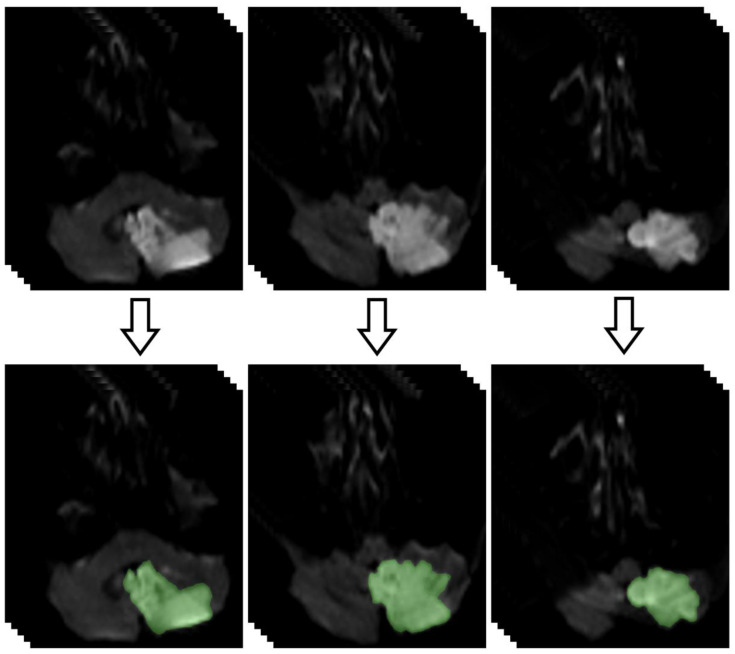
DWI images showing the segmentation of a left cerebellar acute stroke to calculate the total volume of the lesion.
